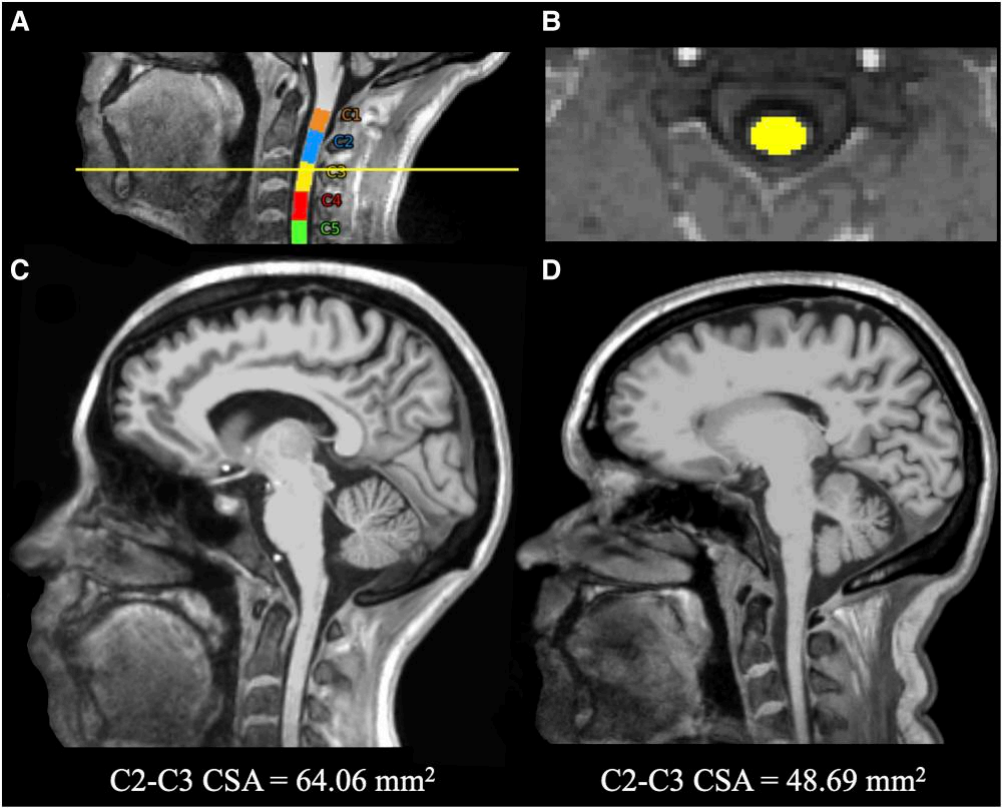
Segmentation of cervical spinal cord and its cross-sectional area estimation. Example of automatic segmentation of the cervical spinal cord on 3T T1-weighted sagittal (A) and axial (B) planes to estimate the C2-C3 CSA, using Spinal Cord Toolbox version 6.1. Different colours in panel A indicate the segmentation of individual cervical spinal cord levels, and the yellow line denotes the axial slice at the C3 level for which the spinal cord segmentation is shown in panel B. Sagittal T1-weighted images show the difference in C2-C3 CSA between a 29-year-old HC (C) and a 49-year-old SPMS patient (D). CSA = cross-sectional area; HC = healthy control; SPMS = secondary progressive multiple sclerosis.
